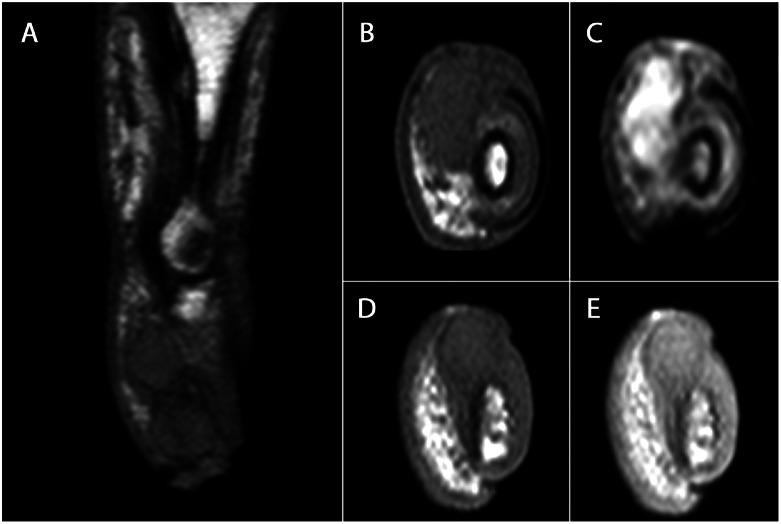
(A) Sagittal T1-weighted MRI of the left third finger (2015) demonstrates 2 adjacent, well-defined soft-tissue nodules in the distal phalanx, each approximately 6 mm in diameter and distinguishable from one another. (B-C) Axial T1-weighted (B) and fluid-sensitive (C) sequences demonstrate the proximal nodule. (D-E) Axial T1-weighted (D) and post-contrast T1-weighted (E) images show the distal nodule with moderate enhancement and evidence of invasion into the underlying phalangeal bone and overlying epidermis.
